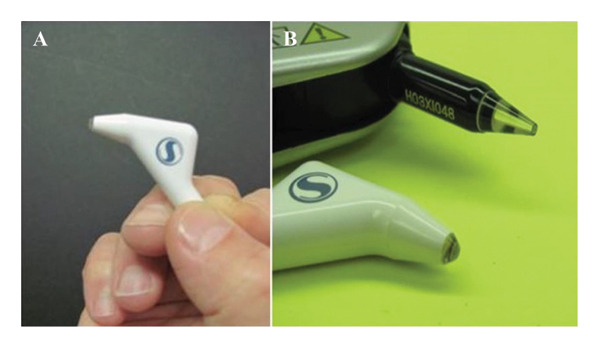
The E‐pach is a new, handheld ultrasonic pachymeter. (A) A handheld E‐pach. (B) The blunt tip of the probe to reduce corneal scratches.
